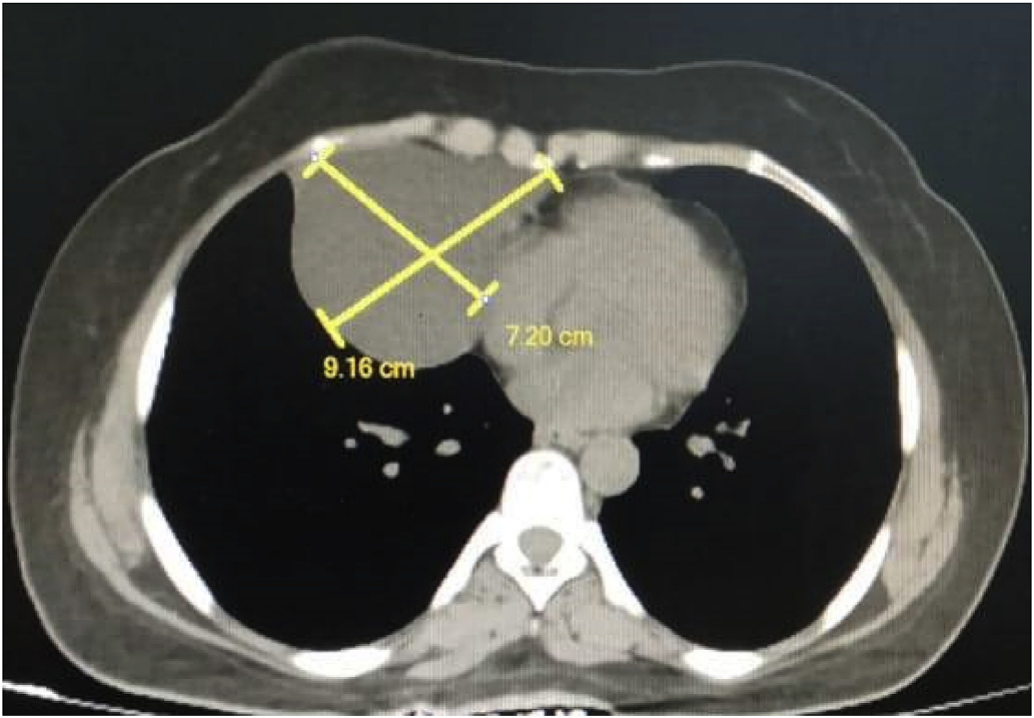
A chest CT scan with contrast shows homogeneous mass occupying the right lower lung lobe Figure 2B: a chest CT scan with contrast shows homogeneous mass measuring 7.2 × 9.16 cm occupying the right lower lung lobe.
